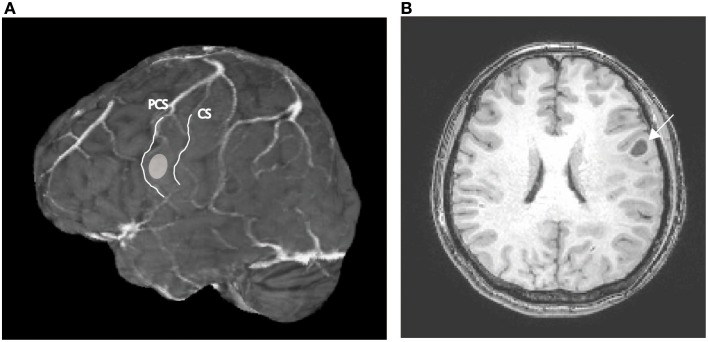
(A) Preoperative MR image of the patient's brain; the ellipsoid indicates the location of the tumor. CS, central sulcus; PCS, precentral sulcus. (B) Axial section of the preoperative T1-weighted MRI; the arrow indicates the tumor in the left precentral gyrus.
